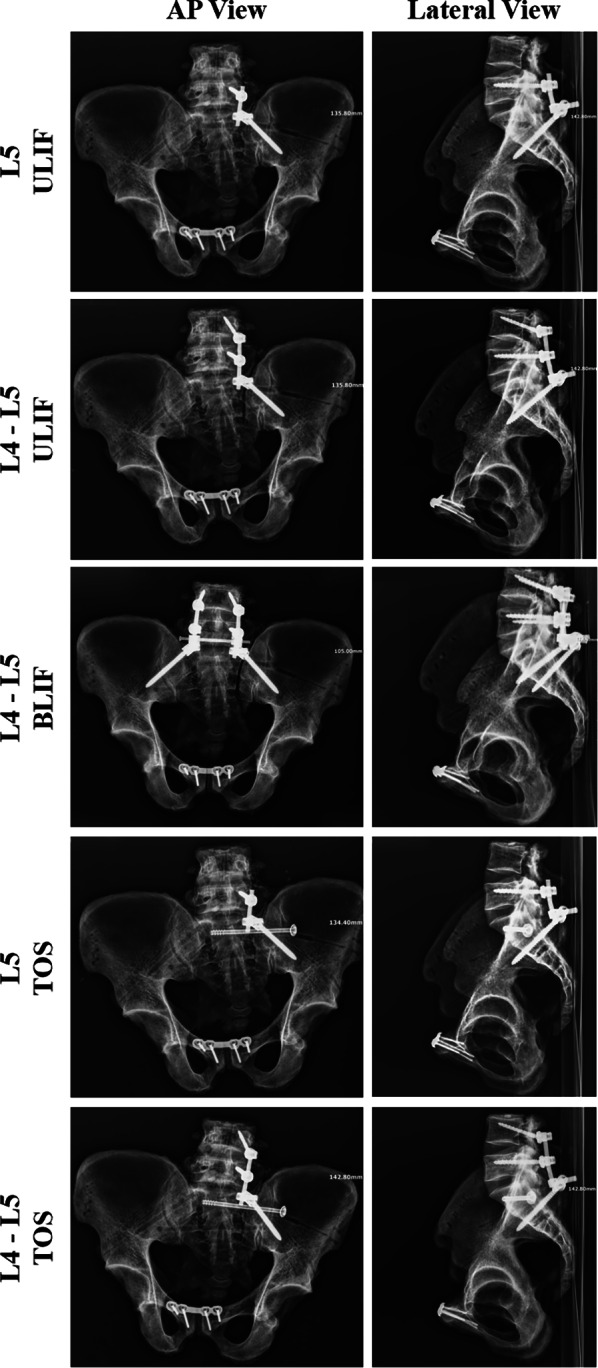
Anteroposterior and lateral pelvic X-rays of L5 ULIF, L4–L5 ULIF, L4–L5 BLIF, L5 TOS, and L4–L5 TOS. *ULIF* unilateral lumbar–iliac fixation, *BLIF* bilateral lumbar–iliac fixation, *TOS* triangular osteosynthesis
